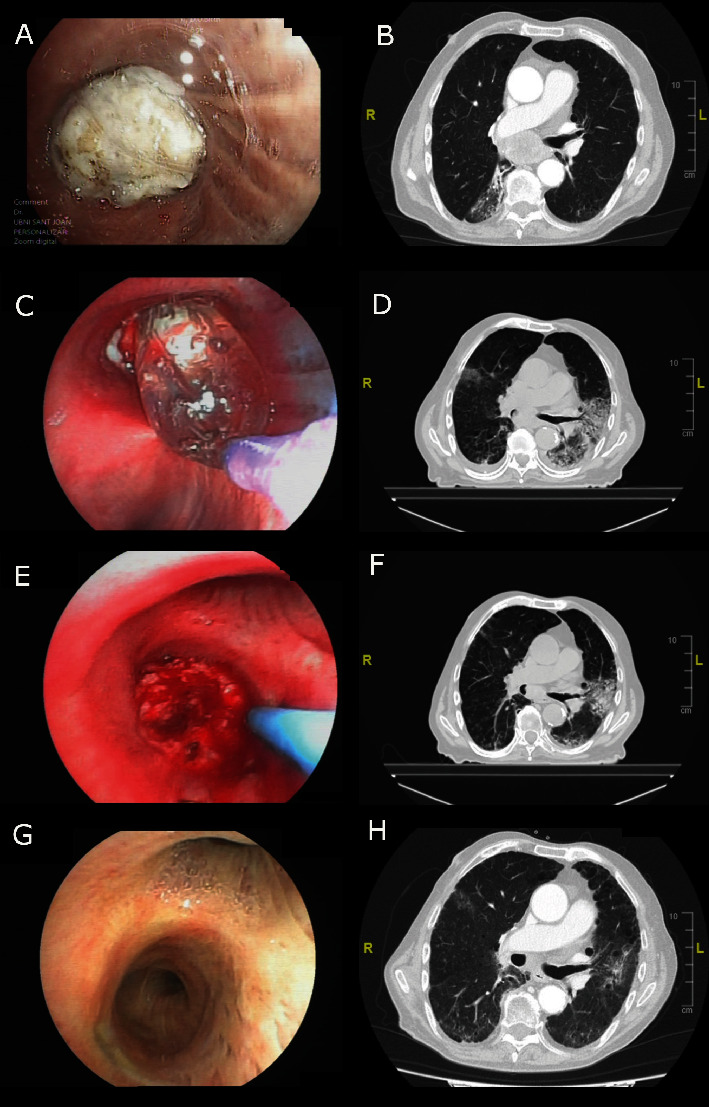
(A) Initial diagnostic bronchoscopy showing a large endobronchial tumor causing a total obstruction of the right main stem bronchus. (B) Initial CT scan with a large subcarinal mass. (C) First therapeutic bronchoscopy with balloon bronchoplasty. (D) CT scan after the start of systemic treatment and bronchoplasty with a slight permeabilization of the bronchial lumen and a decrease in the size of the subcarinal mass. (E) Second therapeutic bronchoscopy when bronchial electrosurgery and argon plasma coagulation (shown in the picture) were applied. (F) Subsequent follow-up CT scan with a recovery of 50% of the lumen of bronchus intermedius and a decrease in the size of the subcarinal mass. (G) Final bronchoscopic evaluation showing total regression of the endobronchial tumor with a preserved bronchial lumen of bronchus intermedius. (H) Final CT scan with a maintained decrease in size of the subcarinal mass.
